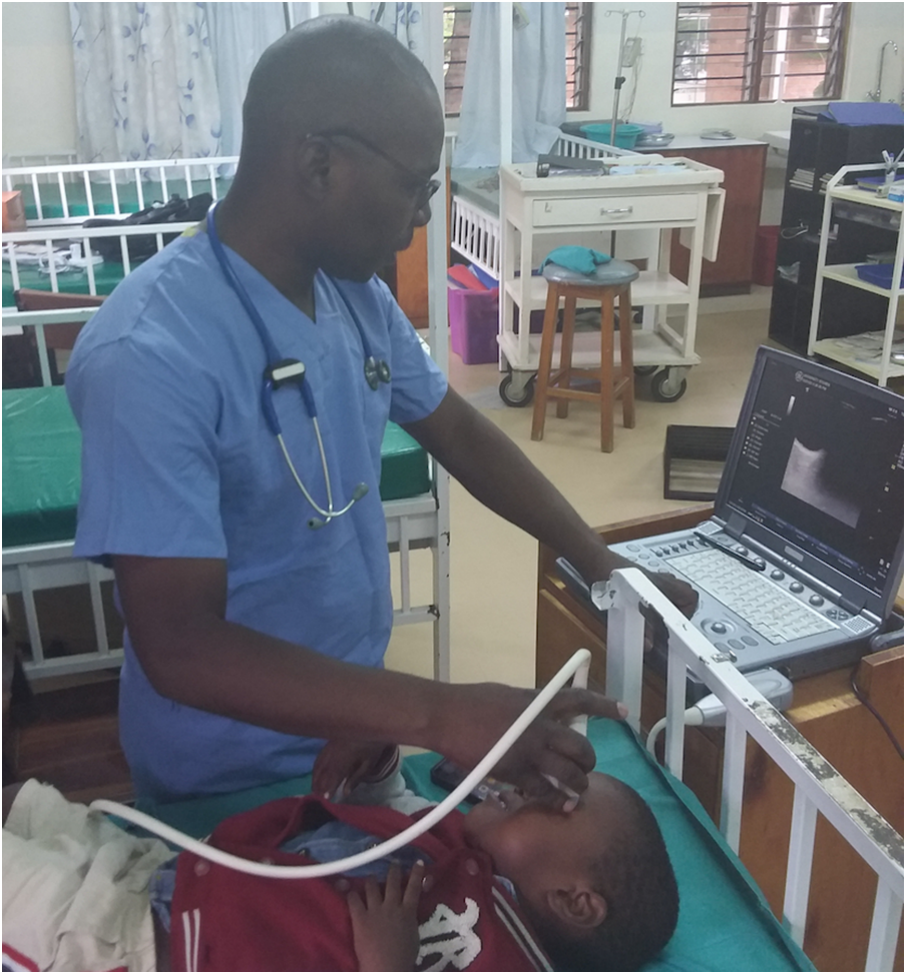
B-mode technique of bedside ultrasound operation to visualize optic nerve sheath and measure diameter.
